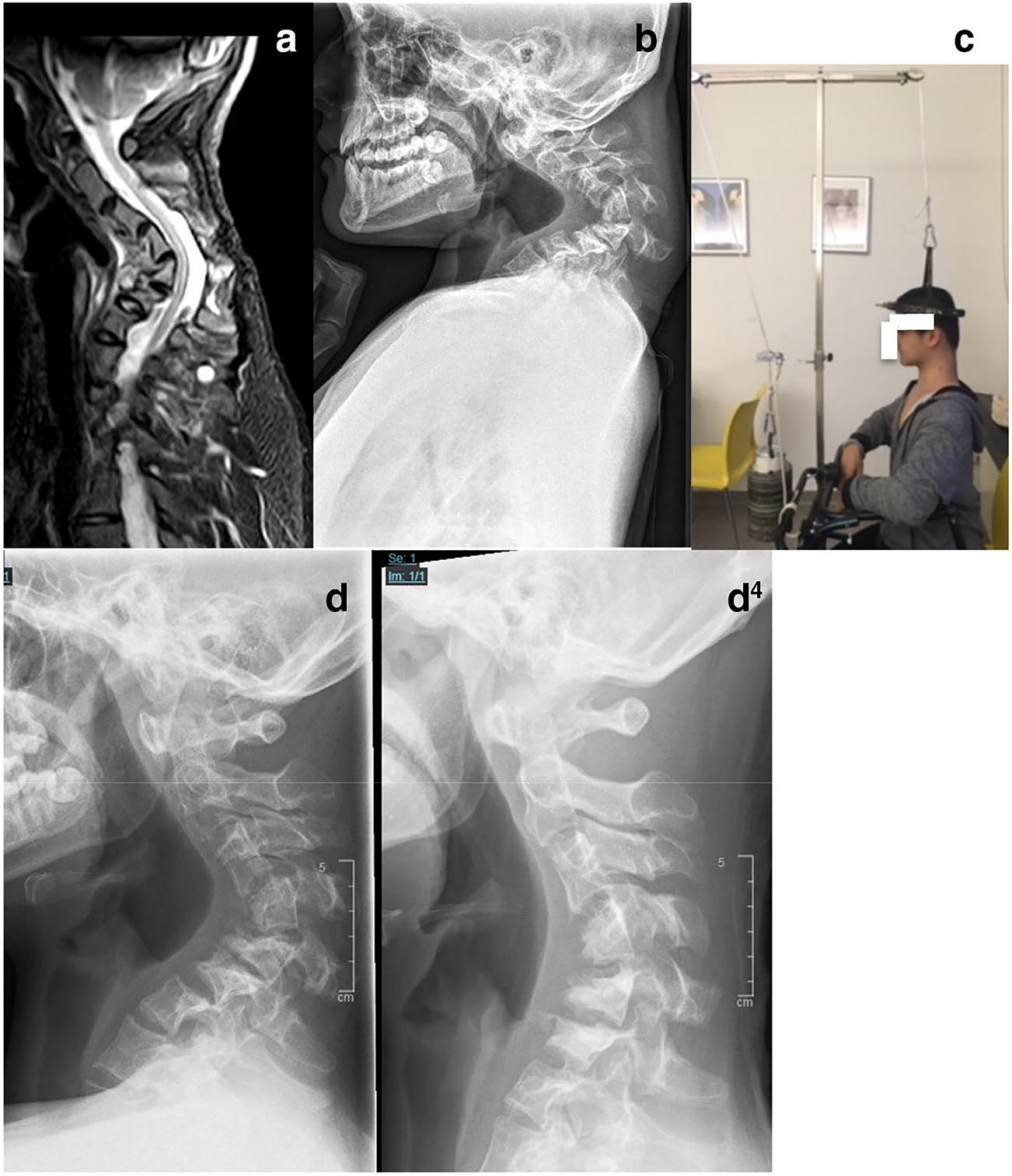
(Case 1) Lateral MRI (a) and plain X-ray films (b) of a 15-year-old patient with NF-1 showing severe kyphosis of the C-spine. Clinical image in preoperative halo-gravity traction (c). X-rays 2 weeks and 4 weeks (d) after the beginning of the HGT. Intraoperative image intensifier images after anterior cage and plate insertion (e, f) and clinical photos of the surgical site (g, h). Postoperative X-rays in a halo jacket (i, j)
